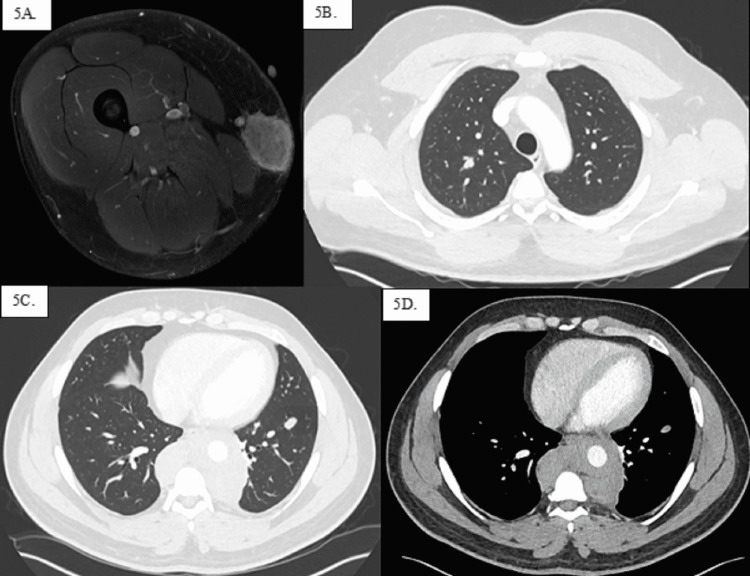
Case 5: Rosai-Dorfman disease systemic involvement A. Magnetic resonance imaging (MRI) with and without contrast showed a 5.1 cm homogenously contrast-enhancing mass within the right medial thigh (axial view). B. Computed tomography (CT) of the chest demonstrated a right upper lobe 1.2 cm non-calcified nodule. C. CT of the chest demonstrated a left lower lobe 1.3 cm solid, non-calcified nodule. D. CT of the chest showed a 10.6 x 9 cm homogenous, confluent paraspinal and posterior mediastinal soft tissue mass that surrounds the aorta without compression, which was concerning for metastatic disease.
